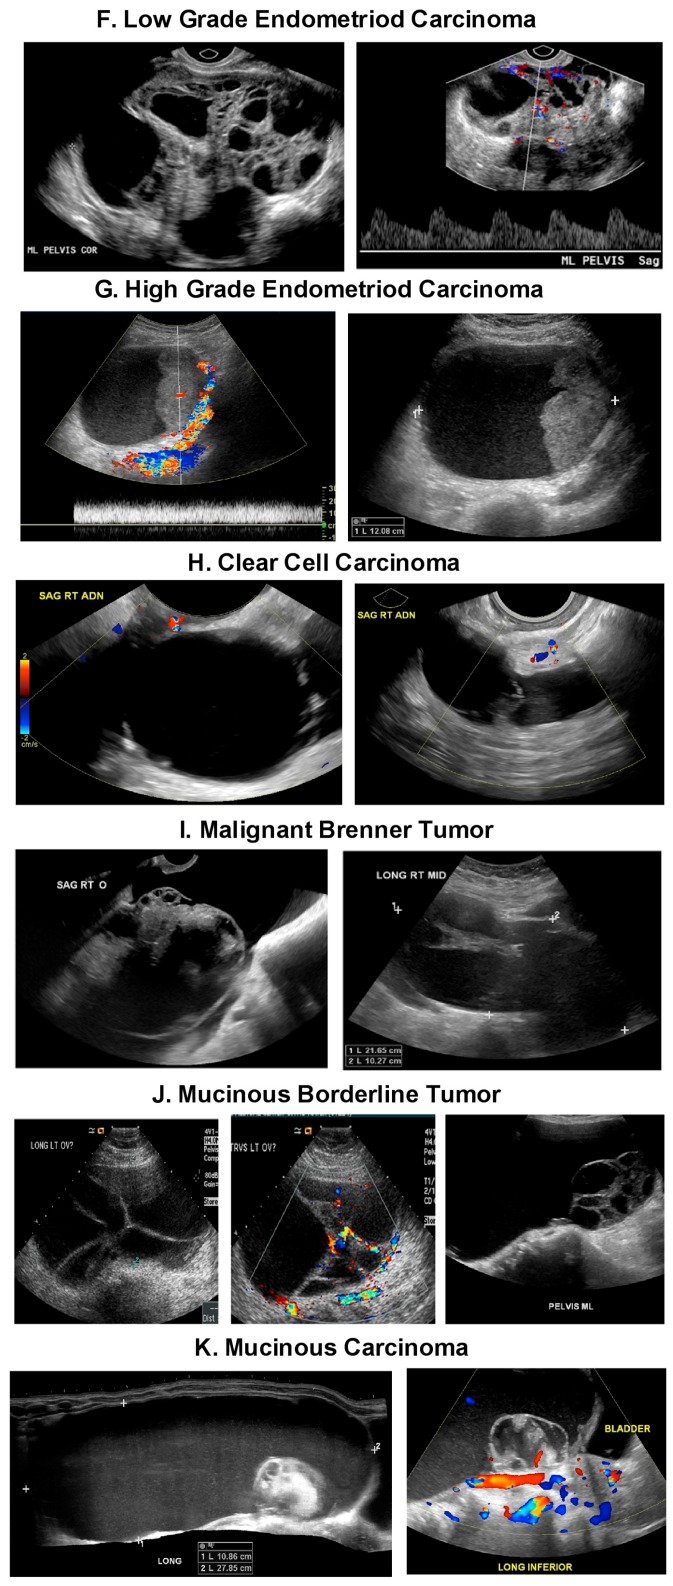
Ultrasonographic appearance of borderline, Type 1 and Type 2 ovarian cancers. (A) Bilateral Serous Borderline Tumor: tumors of low malignant potential (i.e., borderline tumors) account for 15% of all epithelial ovarian cancers. Nearly 75% of these tumors are stage I at the time of diagnosis. They represent a heterogeneous group and occur in younger women with favorable prognosis. However, symptomatic recurrence and death may be found as long as 20 years after therapy in some patients. (B) High Grade Serous Carcinoma (Type 2): serous carcinomas comprise the majority of ovarian carcinomas. Unlike low-grade serous carcinoma, *TP53* mutation occurs in up to 80% of high-grade tumors [17,20]. (C) Undifferentiated Carcinoma (Type 2): about 5% of ovarian cancers are so poorly differentiated and difficult to classify that they are called undifferentiated carcinomas and occur as large, solid hemorrhagic structures with necrosis. (D) Malignant Mixed Mesodermal Tumor (Type 2): occur almost exclusively in postmenopausal women. (E) High grade transitional cell carcinoma (Type 2) is probably not a distinct entity but a poorly differentiated form of serous or endometrioid carcinoma. (F) Low Grade Endometrioid Carcinoma (Type 1): endometriosis a likely precursor of endometrioid carcinoma. (G) High grade Endometriod carcinoma (Type 2) is morphologically indistinguishable from high grade serous carcinoma. (H) Clear Cell Carcinoma (Type 1): as with endometrioid carcinomas, there is a close association between endometriosis and clear cell carcinoma. (I) Malignant Brenner Tumor (Type 1): relatively uncommon neoplasm. Most Brenner tumors are benign, only 2–5% being malignant. (J) Mucinous Borderline Tumor (Type 1): 53.3% of borderline tumors are serous tumors and 42.5% are mucinous tumors (42.5%). (K) Mucinous Carcinoma (Type 1): frequently has a heterogeneous composition with coexisting elements of cystadenoma, stromal microinvasion, noninvasive carcinoma, and invasive carcinoma.
